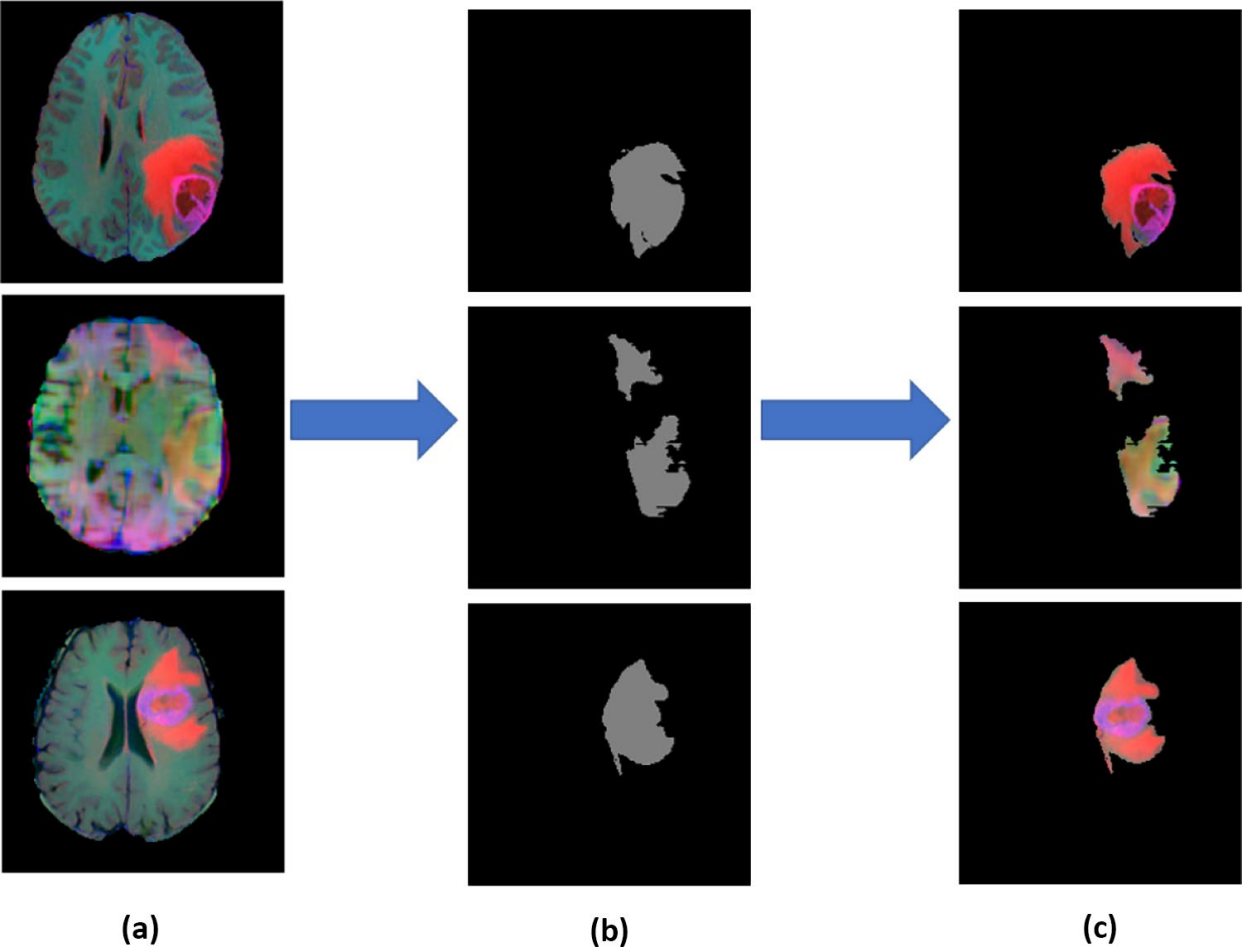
(a) Combined MRI modalities, (b) ROI masks Images and (c) MRI ROI images
